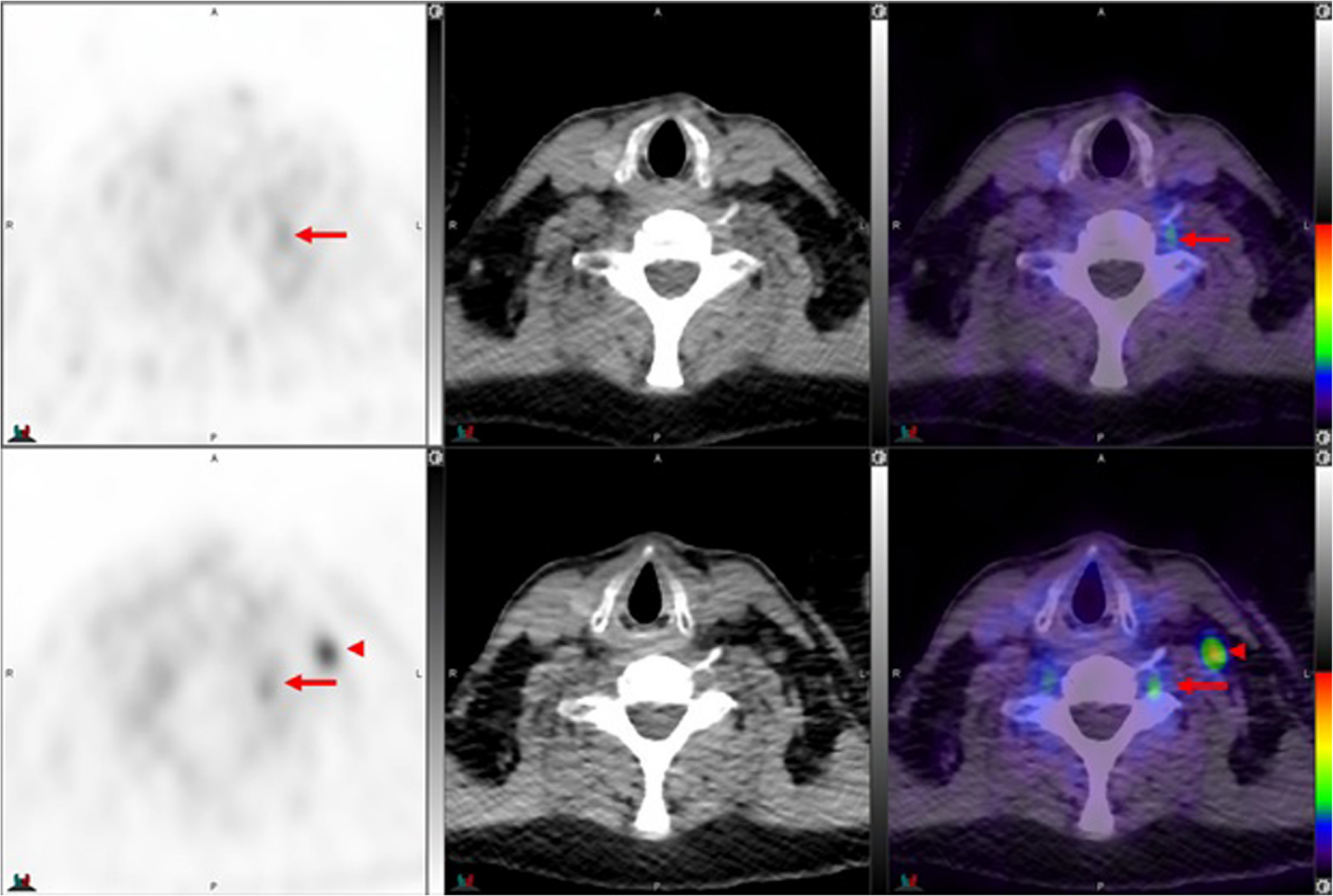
Top panel ^68^Ga-PSMA-11: PET, CT and PET/CT. Left cervical ganglion (arrow) maximum Standard Uptake Value (SUVmax) = 2.2. Lower panel ^18^F –DCFPyL: PET, CT and PET/CT. Left cervical ganglion (arrow) SUVmax = 2.6, Right cervical ganglion SUVmax = 2.3. Of note, ^18^F –DCFPyL shows new left supraclavicular metastases (arrow head). The color intensity has been slightly adjusted to highlight the uptake in the ganglia. There is higher and bilateral lower cervical ganglia uptake with ^18^F –DCFPyL. By CT, there is typically no anatomic structure detectable in the region of cervical ganglia; however, location, bilaterality and multiplicity would provide clues
